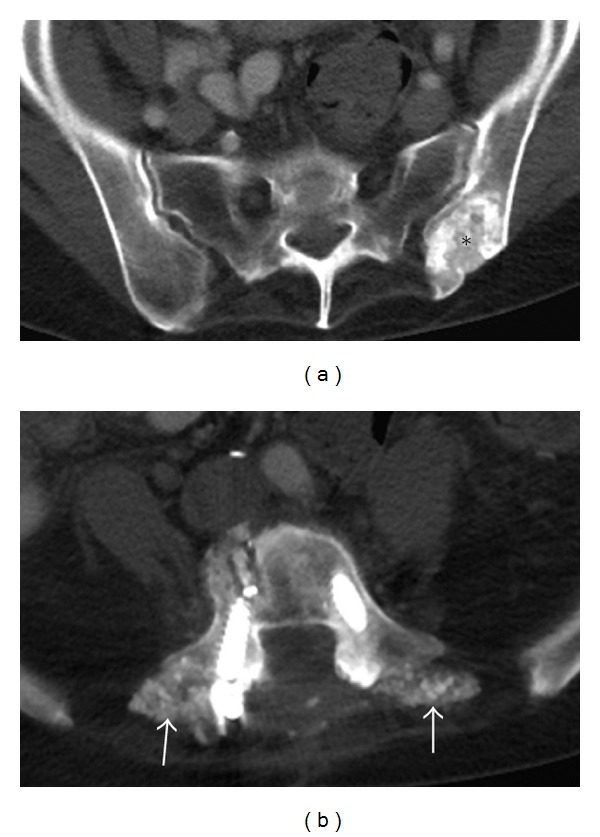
Bone graft donor site. Axial CT (a) demonstrates an aggressive appearing lesion in the left ilium, in a classic location of bone graft donor site. In this case bone graft material (arrows) was used in posterior spinal fusion surgery as shown in the axial CT (b).
